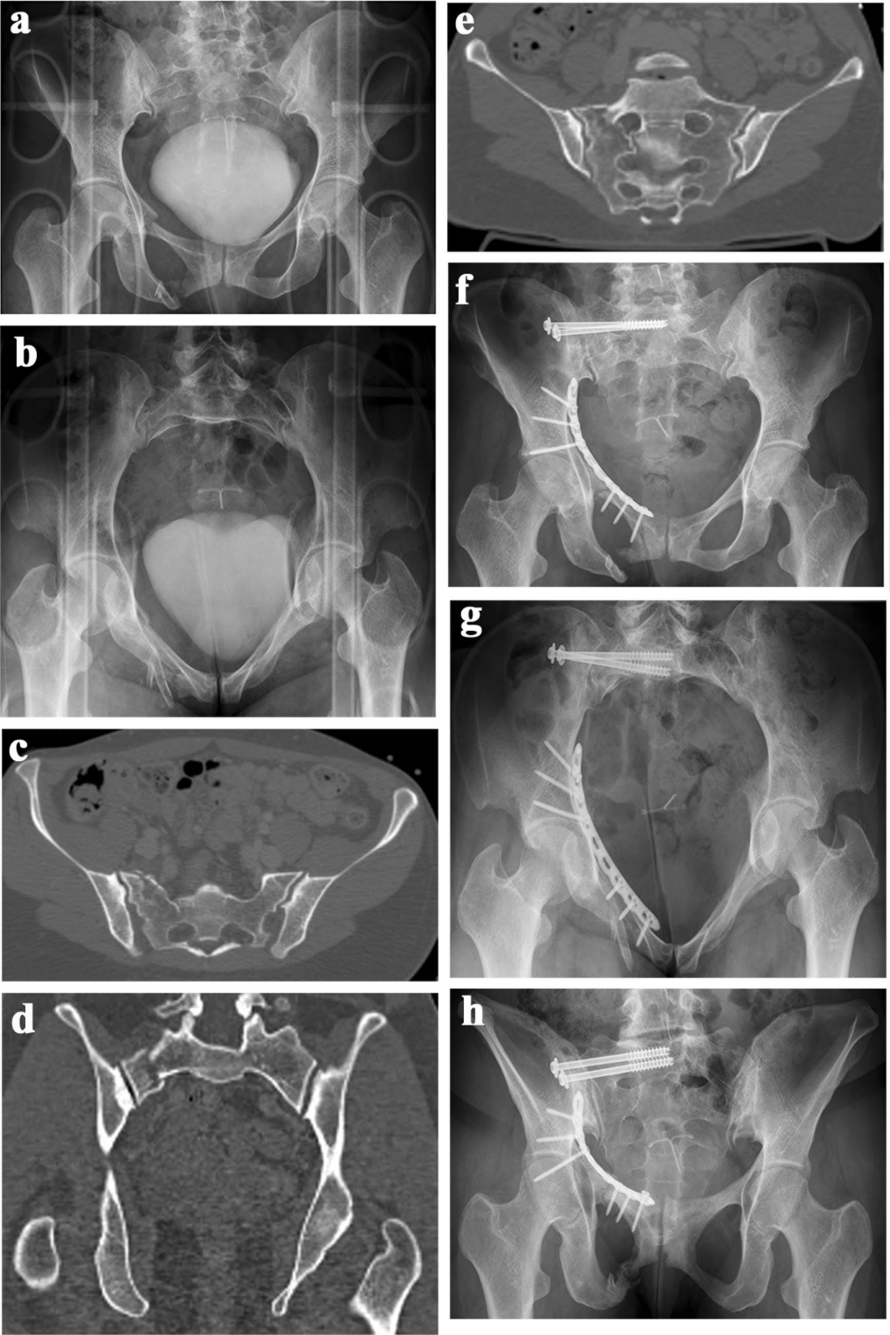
a 44-year-old female suffered an unstable pelvic fracture after a fall from 7 m of height. AP-pelvic overview shows displaced superior and inferior pubic rami fractures. b Pelvic inlet view shows a right-sided sacral ala fracture and the right-sided superior and inferior pubic rami fractures. c Axial CT-slice through the posterior pelvis. There is a right-sided sacral fracture. d Coronal CT-slice through the posterior pelvis. The disploaced right.sided sacral fracture is clearly visible. e CT-reconstruction through the longitudinal axis of the sacrum. The sacral fracture runs through the neuroforamina S1 and S2. Dysmorphism of the upper part of the sacrum can be recognized. f Postoperative AP-view of the pelvis. The sacral fracture was stabilized with two iliosacral screws in S1, the superior pubic ramus fracture was stabilized with a buttress plate through the modified Stoppa approach. There were no postoperative problems. No postoperative CT scan was performed. g Pelvic inlet view. h Pelvic outlet view
